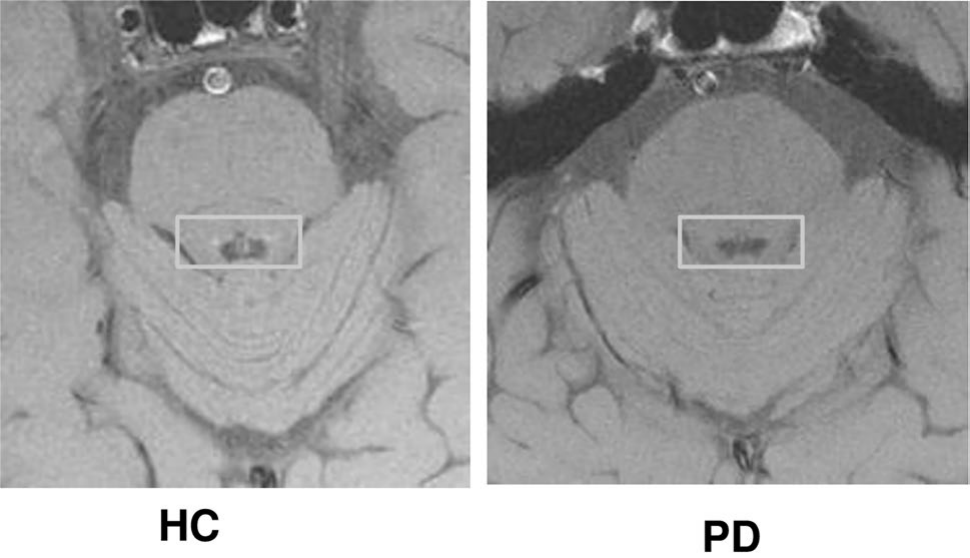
Representative locus coeruleus (LC)–magnetic resonance imaging (LC-MRI) images from PD and healthy controls (HC). The figure shows representative images from 2D-FSE T1-weighted sequence in native space (before post-acquisition LC data extraction, see methods) in corresponding slices at the level of the pons, from one PD and one HC. In each slice, a box insert shows the region where the two LC are placed (visualized just laterally to the median plane, below the floor of the fourth ventricle). In PD, there is a marked decrease in LC-related intensity compared with HC
